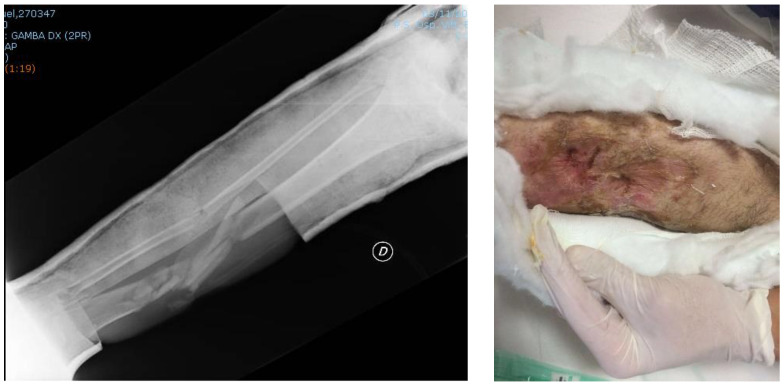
Radiograph of the right leg and image of the soft tissue in November 2016.
